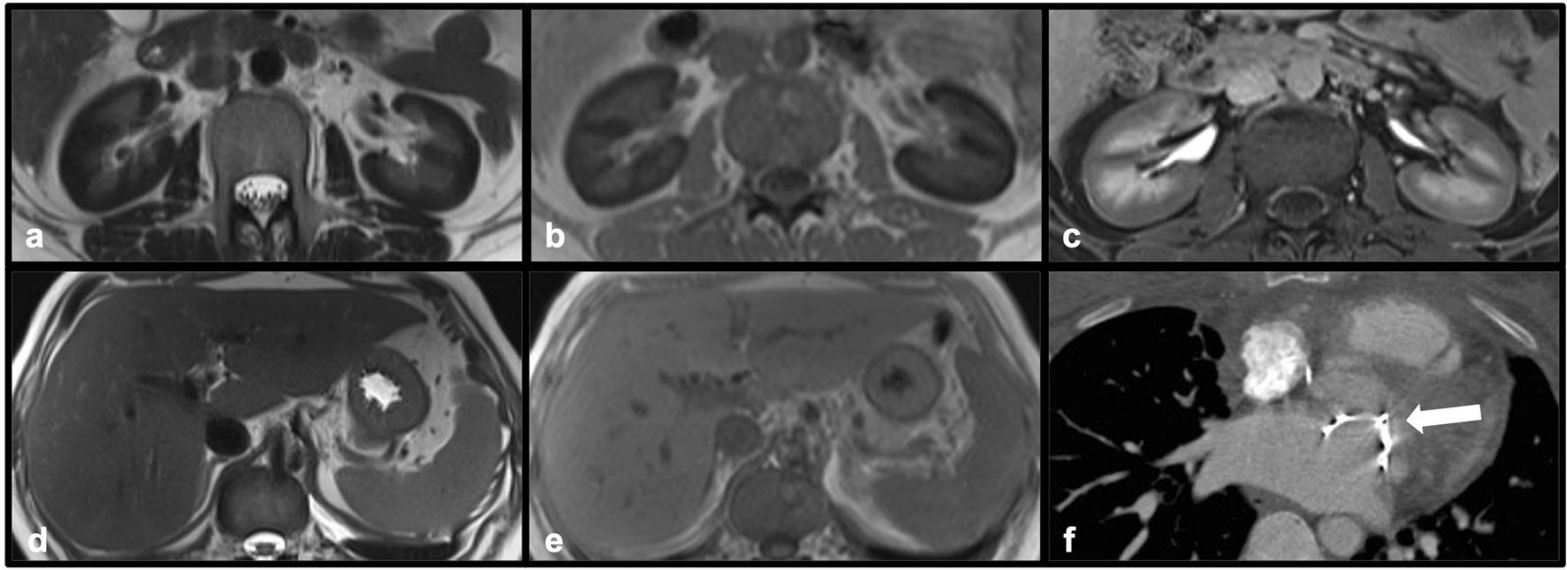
Axial T2 (a), GRE (b) and post-contrast T1-VIBE (c) MR images demonstrate diffusely decreased signal of the renal cortex bilaterally consistent with siderosis. Axial T2 (d) and GRE (e) demonstrate normal signal intensity of the liver and spleen. The prosthetic mitral valve (white arrow) resulting in hemolysis can be seen on axial contrast-enhanced CT (f), the source of renal cortical siderosis in this case
